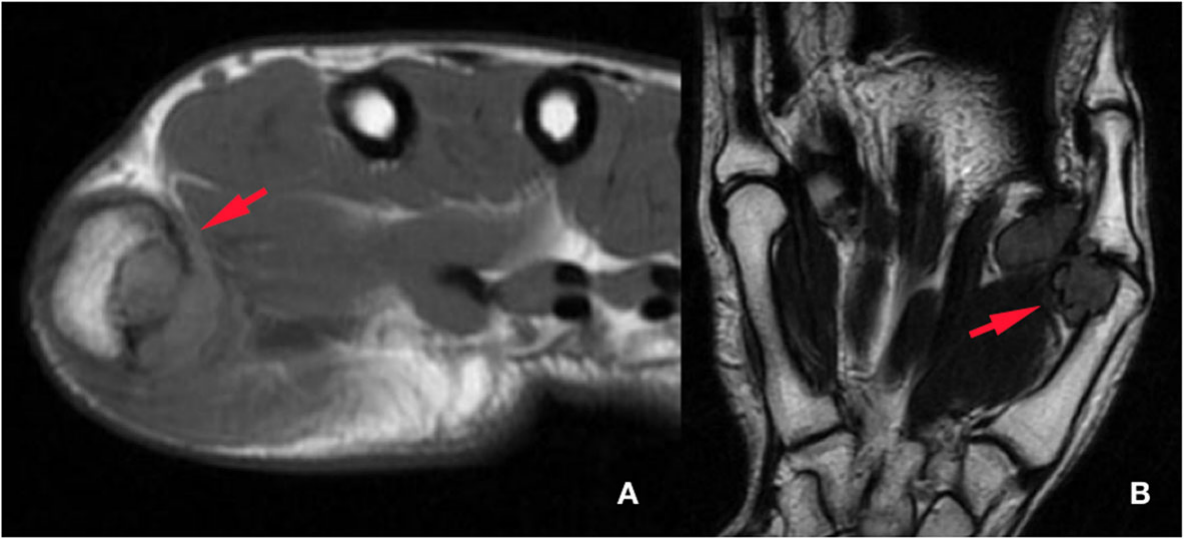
Axial (a) and coronal (b) MRI shows that GCTTS of thumb ray has penetrated into metacarpal head and metacarpophalangeal joint
